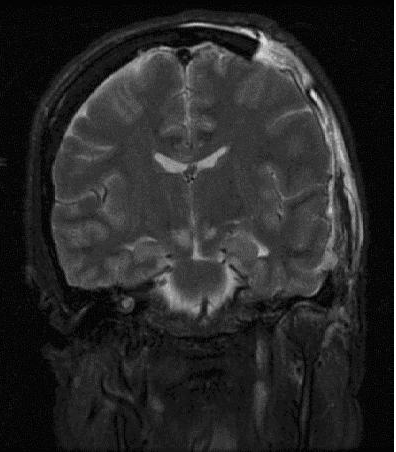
Coronal MRI T2 FLAIR sequence showing herniation injury.
